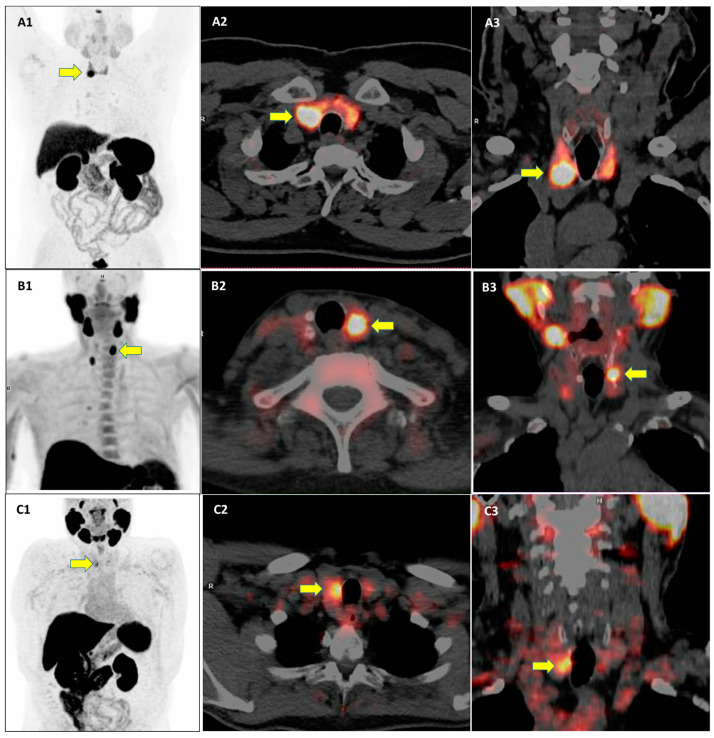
Examples of three cases of thyroid incidental findings (yellow arrows) at PET/CT using different PET radiopharmaceuticals. PET/CT images using [^68^Ga]Ga-DOTA-peptides (A1–A3), radiolabeled choline (B1–B3), and radiolabeled prostate-specific membrane antigen (C1–C3) are provided.
